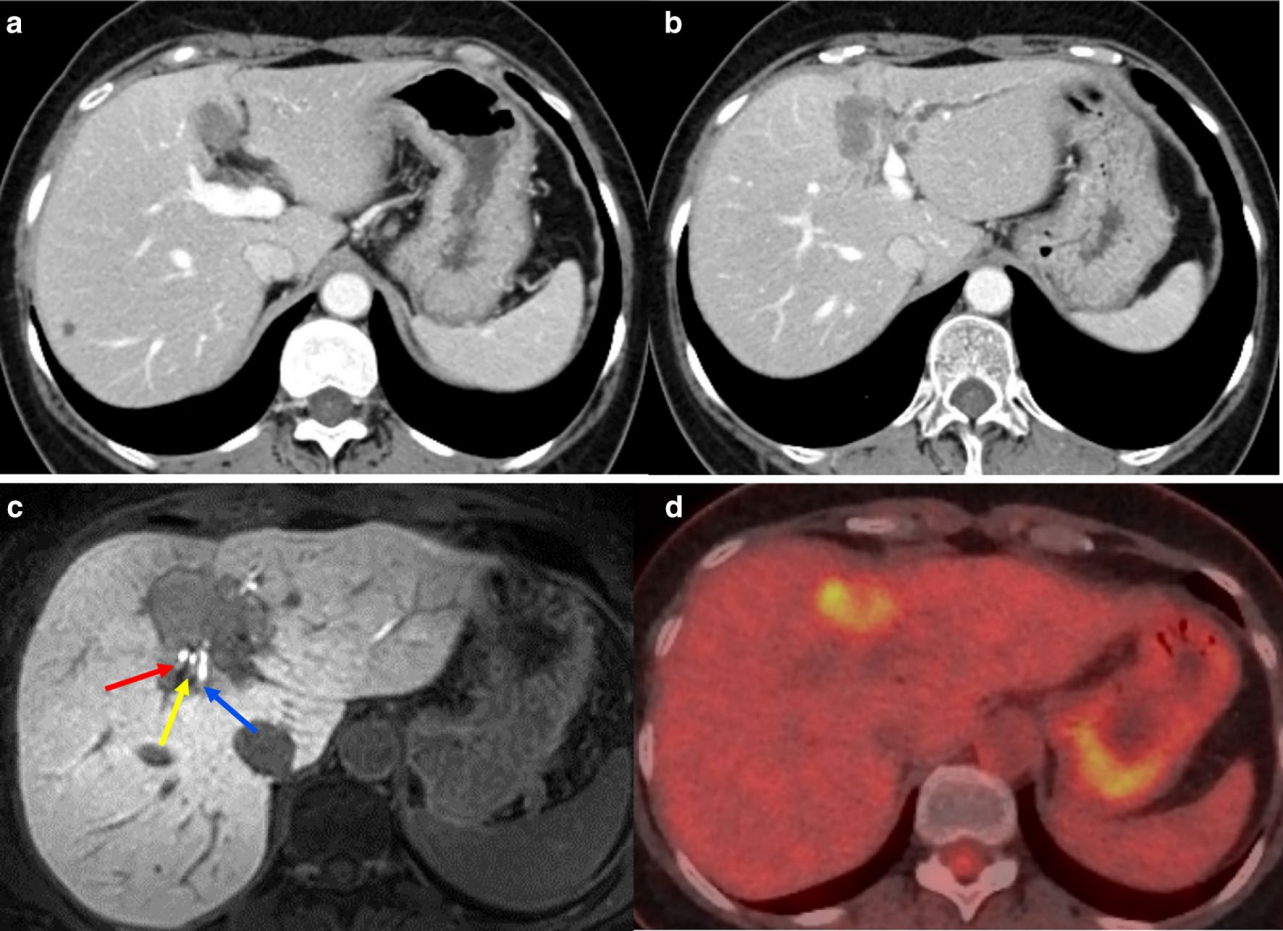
Images after total 10 courses of the combination chemotherapy with gemcitabine plus cisplatin. a, b Contrast-enhanced abdominal CT showed the tumor had decreased to 38 × 35 mm (42% reduction). c The tumor was close to the hilar plate. But no findings of invasion to right anterior dorsal bile duct (red arrow), right anterior ventral bile duct (yellow arrow) and right posterior hepatic duct (blue arrow) were observed magnetic resonance imaging. d FDG-PET/CT showed FDG uptake of tumor had decreased SUV max 6.5
